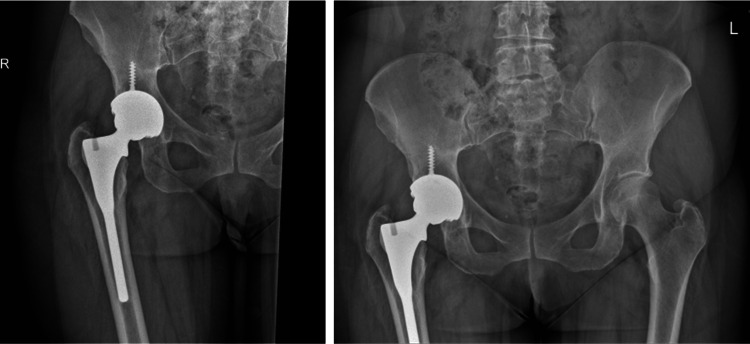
Pre-revision radiographic images. Radiographs demonstrate a right primary THA with a JRI Furlong cementless stem, a 32 mm ceramic head, and a JRI cementless CSF shell with a ceramic liner. THA, total hip arthroplasty
